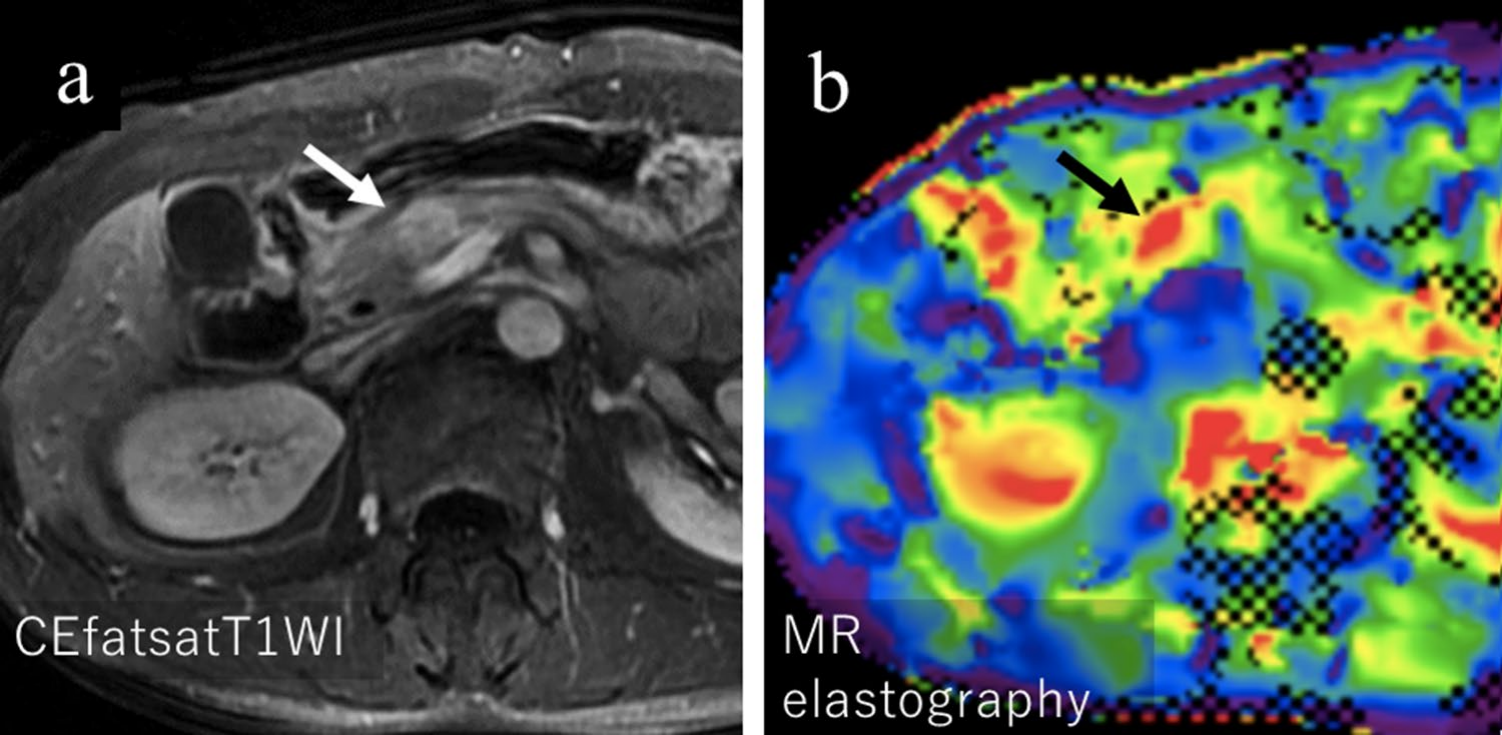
A male in his 50 s with IgG4-related autoimmune pancreatitis (AIP) associated with pathologically proven desmoplastic inflammatory pseudotumor. a Contrast-enhanced T1-weighted image showing delayed positive enhancement of the lesion in the pancreatic head (arrow). b A color-coded stiffness map shows a high stiffness of 6.2 kPa in the lesion (arrow), higher than previously reported values for AIP
